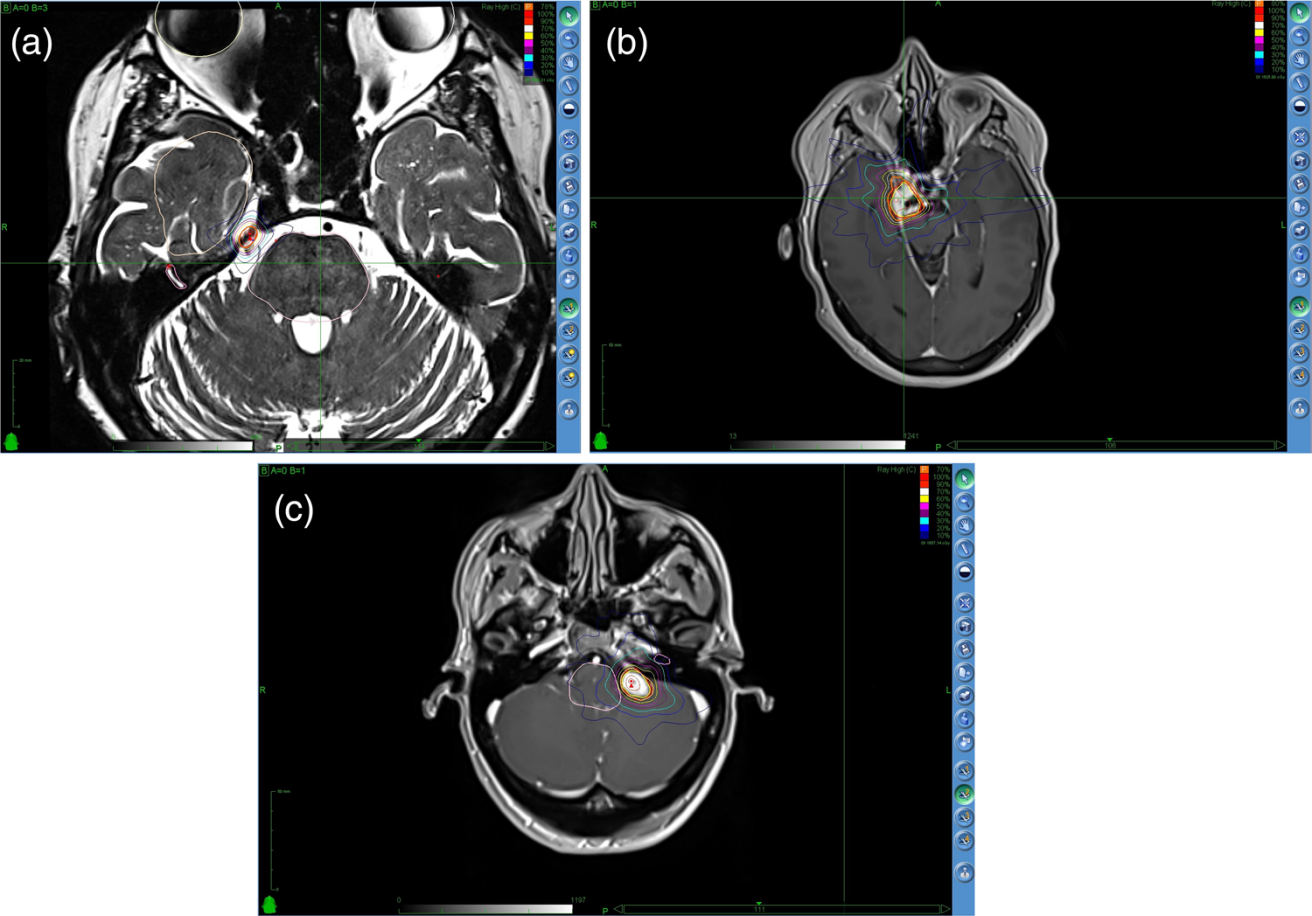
The isodose distribution in the transverse MRI plane from a representative (a) trigeminal neuralgia, (b) meningioma, and (c) acoustic neuroma CyberKnife treatment plan. These treatment plans were prescribed to the (a) 78%, (b) 80%, and (c) 70% isodose line.
